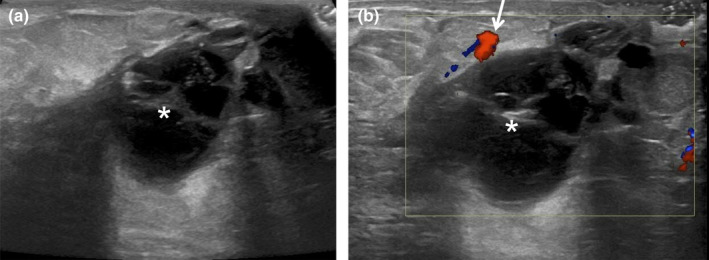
73‐year‐old woman with increased pain and bruising after an ultrasound‐guided biopsy, which was performed with a 9‐gauge vacuum‐assisted device. Ultrasound of the right breast at 9 o'clock 4 cm from the nipple shows a complex cystic collection measuring 3 cm (asterisk), consistent with a post‐biopsy haematoma (a). Colour Doppler imaging shows a single prominent adjacent vessel (arrow) without active bleeding or pseudoaneurysm (b). The breast/chest was wrapped in a compression bandage and instructed to limit activity and return to care if symptoms worsened.
